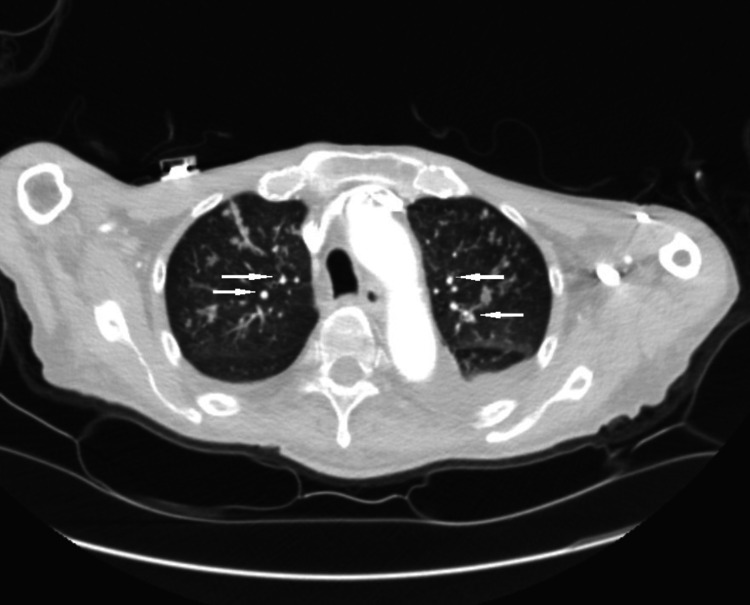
CT scan of the chest with contrast revealed bilateral, irregular nodular densities (white arrows)
